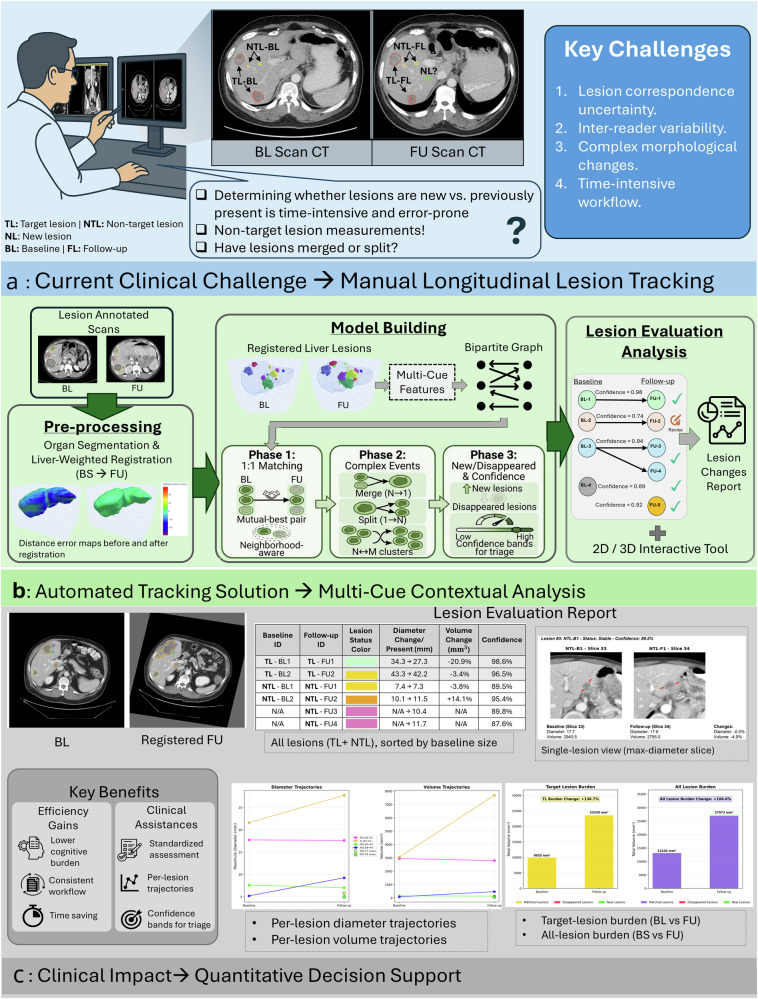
Overview of the proposed longitudinal lesion-tracking framework. a Manual tracking of colorectal liver metastases across serial CT scans is time-intensive, limited to a small number of target lesions, and subject to inter-reader variability, particularly in high-burden disease. b The proposed automated system applies affine liver-weighted registration, multi-cue feature extraction (including spatial, morphologic, and volumetric metrics with intra- and inter-timepoint neighborhood context), and graph-based bipartite matching to generate confidence-scored lesion correspondences. Complex events such as lesion split and merge are explicitly identified. c Outputs include two-dimensional (2D) and interactive three-dimensional (3D) lesion visualizations, confidence-based lesion evaluation reports, lesion-level trajectories, and whole-liver tumor burden metrics, supporting efficient, standardized, and explainable longitudinal assessment
